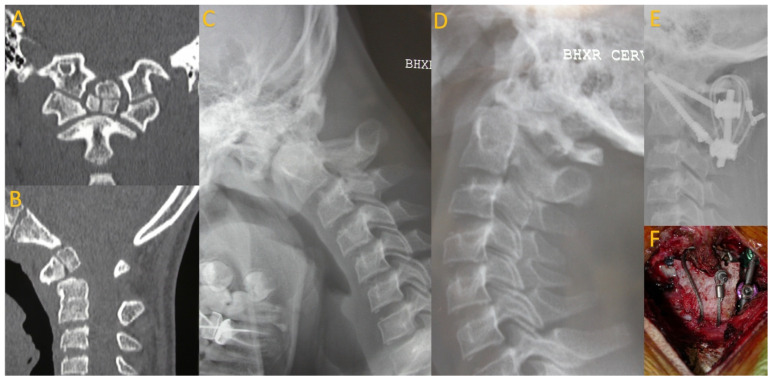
A 16-year-old boxer who presented after developing transient paraplegia while boxing. CT imaging revealed os odontoideum (A,B) with hypermobility on flexion and extension cervical X-rays (C,D). He underwent posterior C1–2 fusion with instrumentation, wiring, and allograft (E,F).
